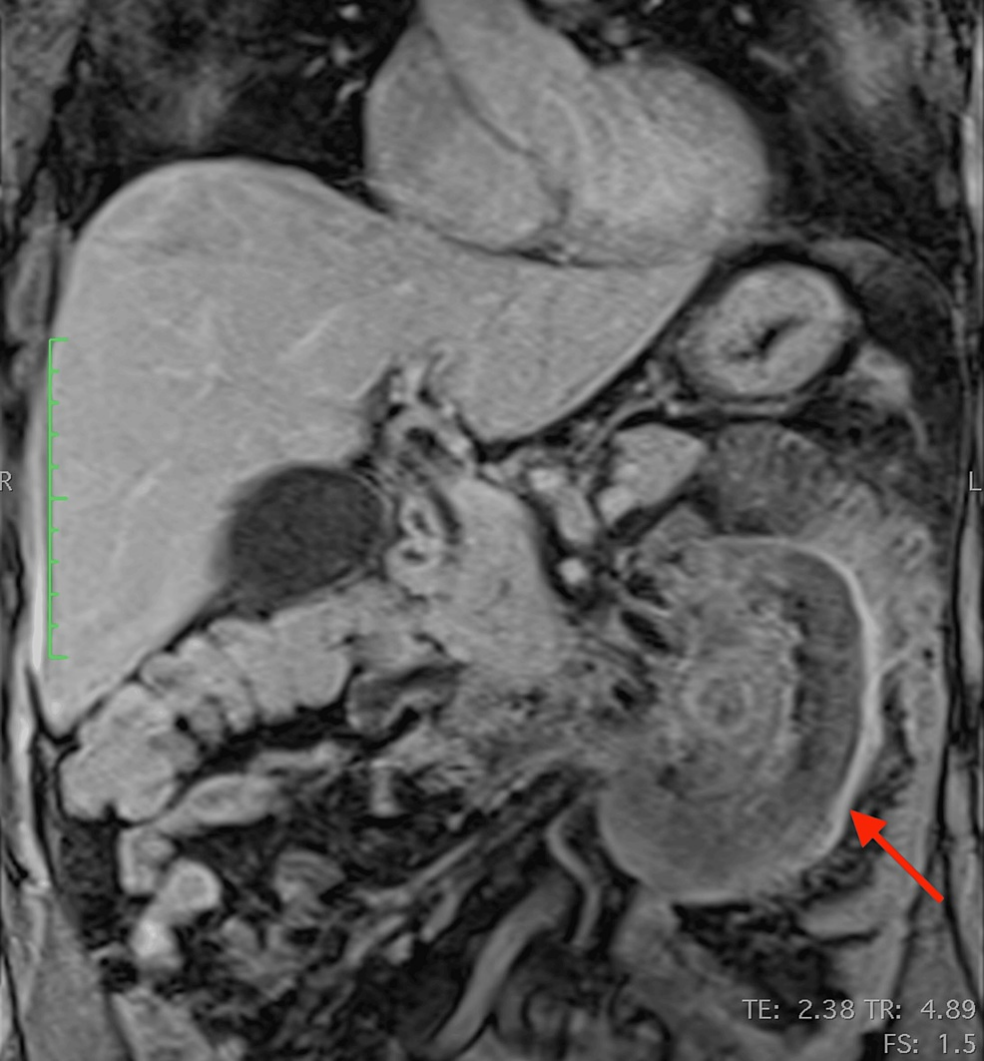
Coronal MRI T1-weighted image, with intravenous contrast infusion Note the peripheral signal enhancement after intravenous contrast infusion MRI: magnetic resonance imaging
